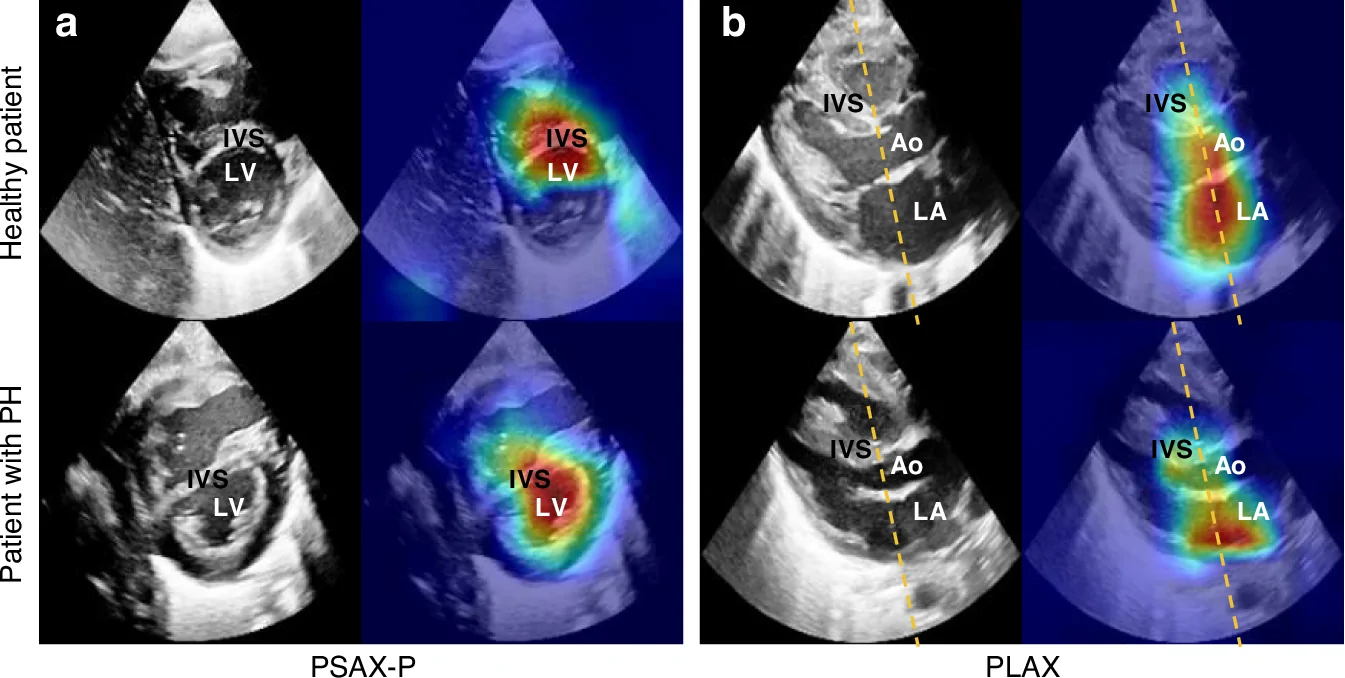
ECHO frames of healthy and patient with PH (left), as well as the IP-PHN saliency maps (right). Panel (a) shows the PSAX-P, parasternal short axis  view, and (b) the PLAX, parasternal long axis view. The yellow line shows how the M-mode for the LA: Ao measurement is extracted. The highlighted pixels feature crucial cardiac structures. LV left ventricle, IVS interventricular septum, LA left atrium, AO aortic root.
